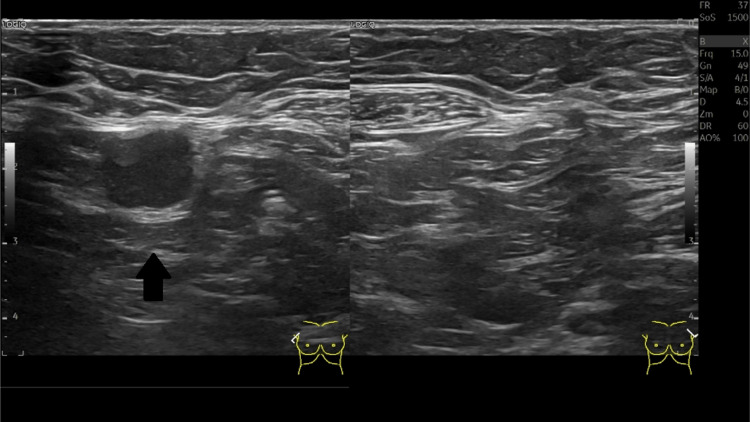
Ultrasonography of the right axillary lymphadenopathy
